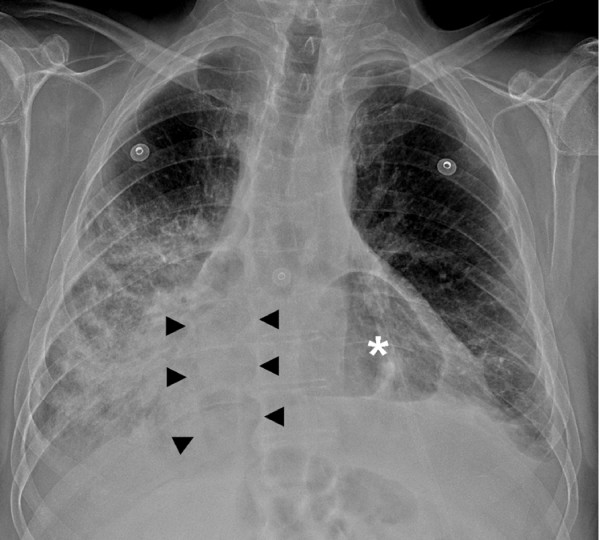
Chest Radiograph. Chest radiograph demonstrating the stomach bubble (white asterisk) projecting over the heart and a portion of the transverse colon that communicates with the abdominal cavity superimposed over the right heart border (black arrowheads).
